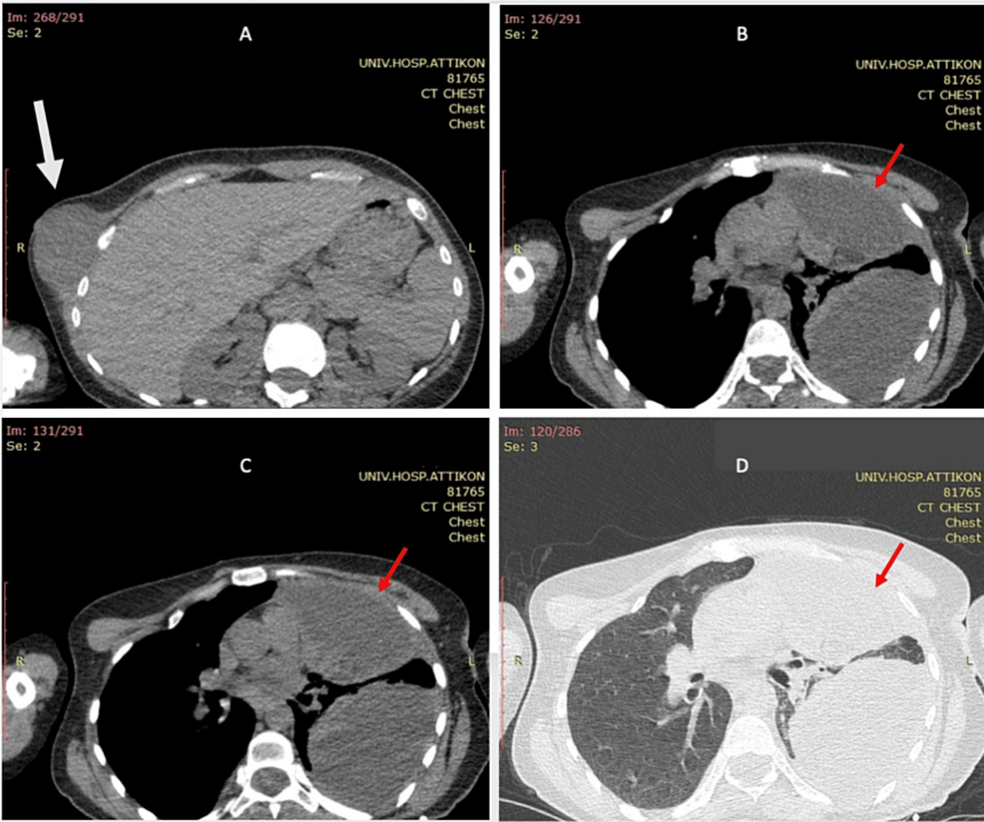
CT scan showing (A) well-defined hypodense large subcutaneous mass arising from the right anterior chest wall (grey arrow) and (B-D) large mediastinal mass anteriorly to the LUL with size 10.9 x 7.9 cm consistent with soft and fat tissue densities and thickening of its wall (red arrows). Increase in mediastinal shift to the right and small to moderate right sided pleural effusion. Presence of lesions with homogenous density in the right pleura with paravertebral location and widening of the right intravertebral spaces (T8-T10) indicating of a neuroma. Small atelectasis at the RLL, no pericardial effusion, lymph node enlargement in the mediastinum and axillary region. CT: Computer Tomography; LUL: Left upper lobe; RLL: Right lower lobe.
